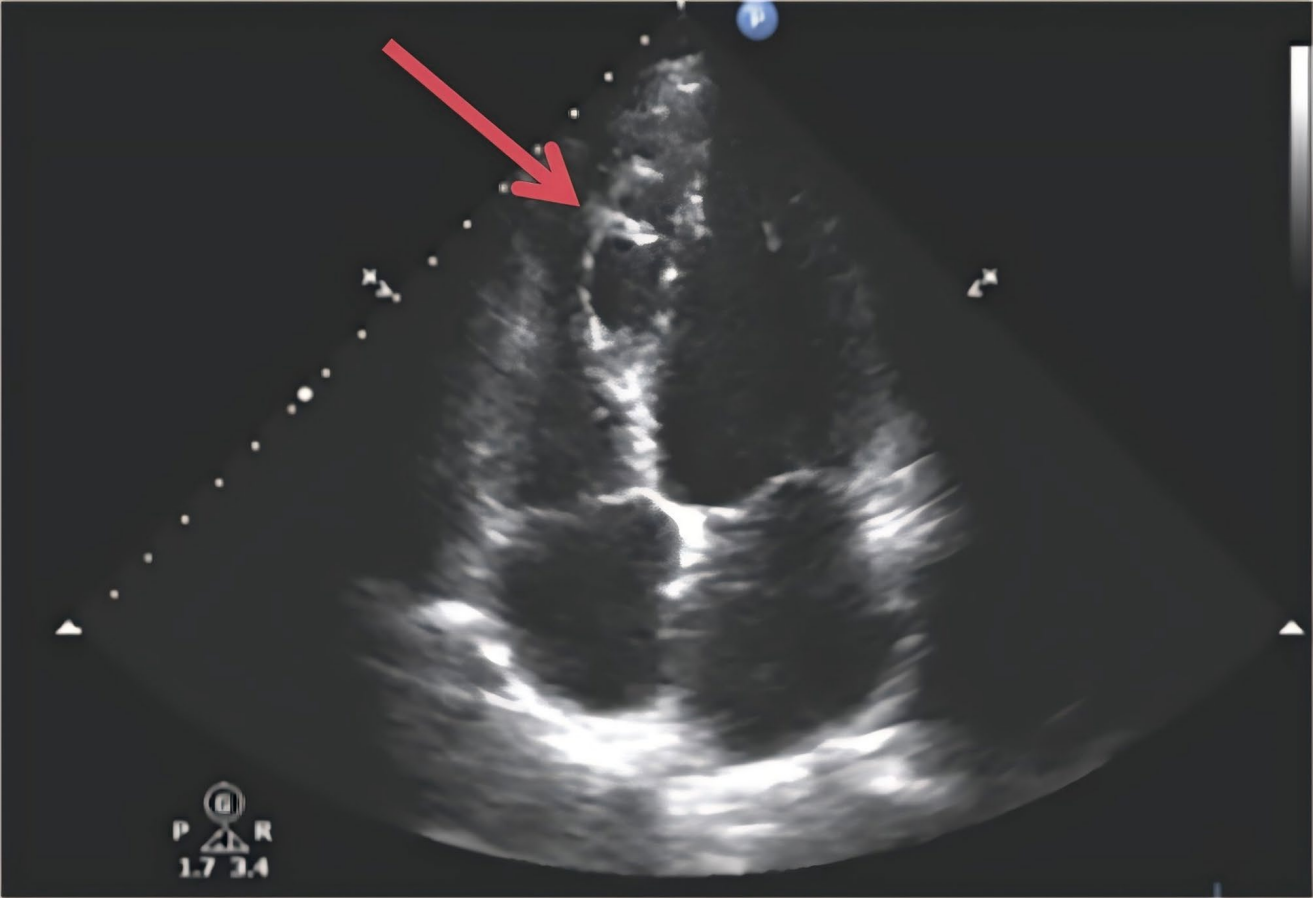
A cardiac echocardiogram showing ventricular septum-inferior wall dissecting aneurysm formation. An irregular anechoic chamber (18 mm wide) communicating with the ventricular cavity was detected between the lower segment of the interventricular septum and the inferior wall
